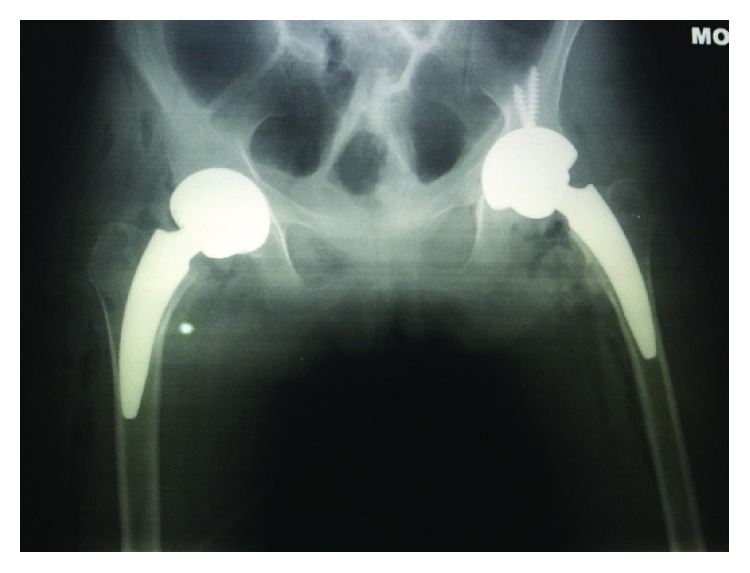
Postoperative plain radiograph of bipolar short-stemmed total hip replacements.
